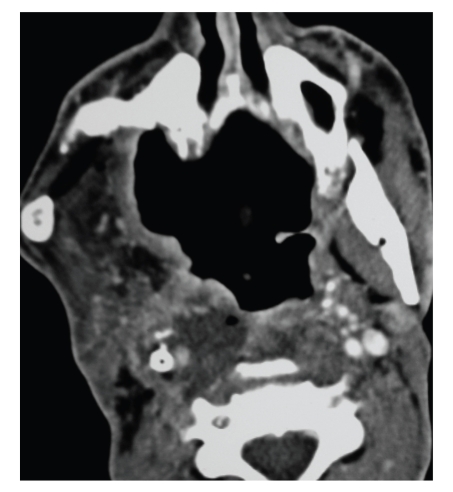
Contrast Enhanced computed tomography scan of the neck showing the right carotid stent occluded with no blood flow in the lumen. The carotid is surrounded by the ulcerative mass.
